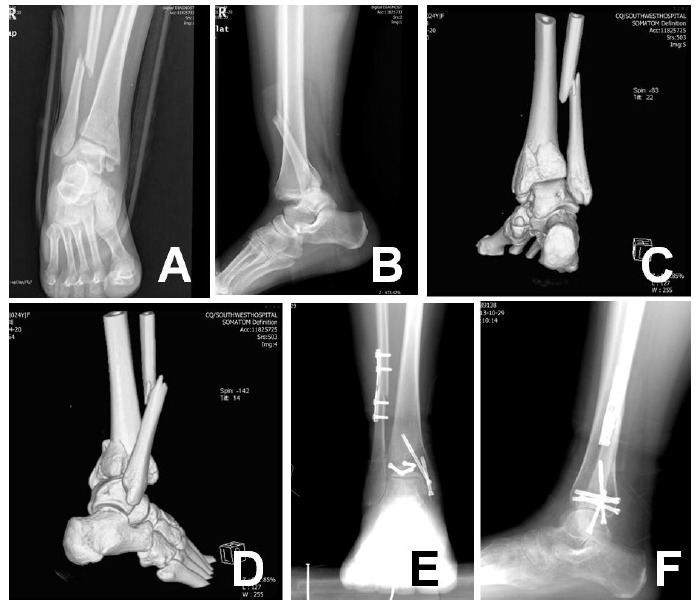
Case 1: (A and B) Anteroposterior and lateral radiographs showing a displaced trimalleolar fracture, (C and D) Three-Dimensional Computed Tomography, (E and F) Postoperative anteroposterior and lateral radiographs.
